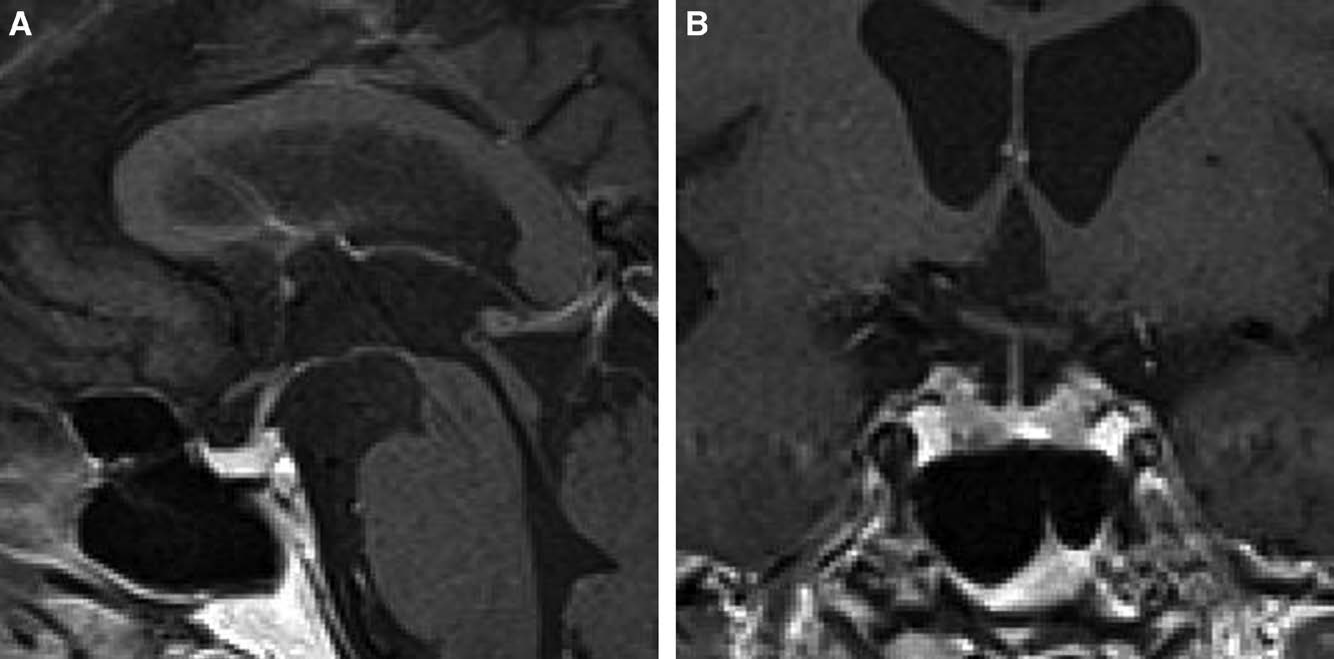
Brain magnetic resonance imaging (MRI) on d 20. (A) Sagittal gadolinium-enhanced T1-weighted MRI. (B) Coronal gadolinium-enhanced T1-weighted MRI.
